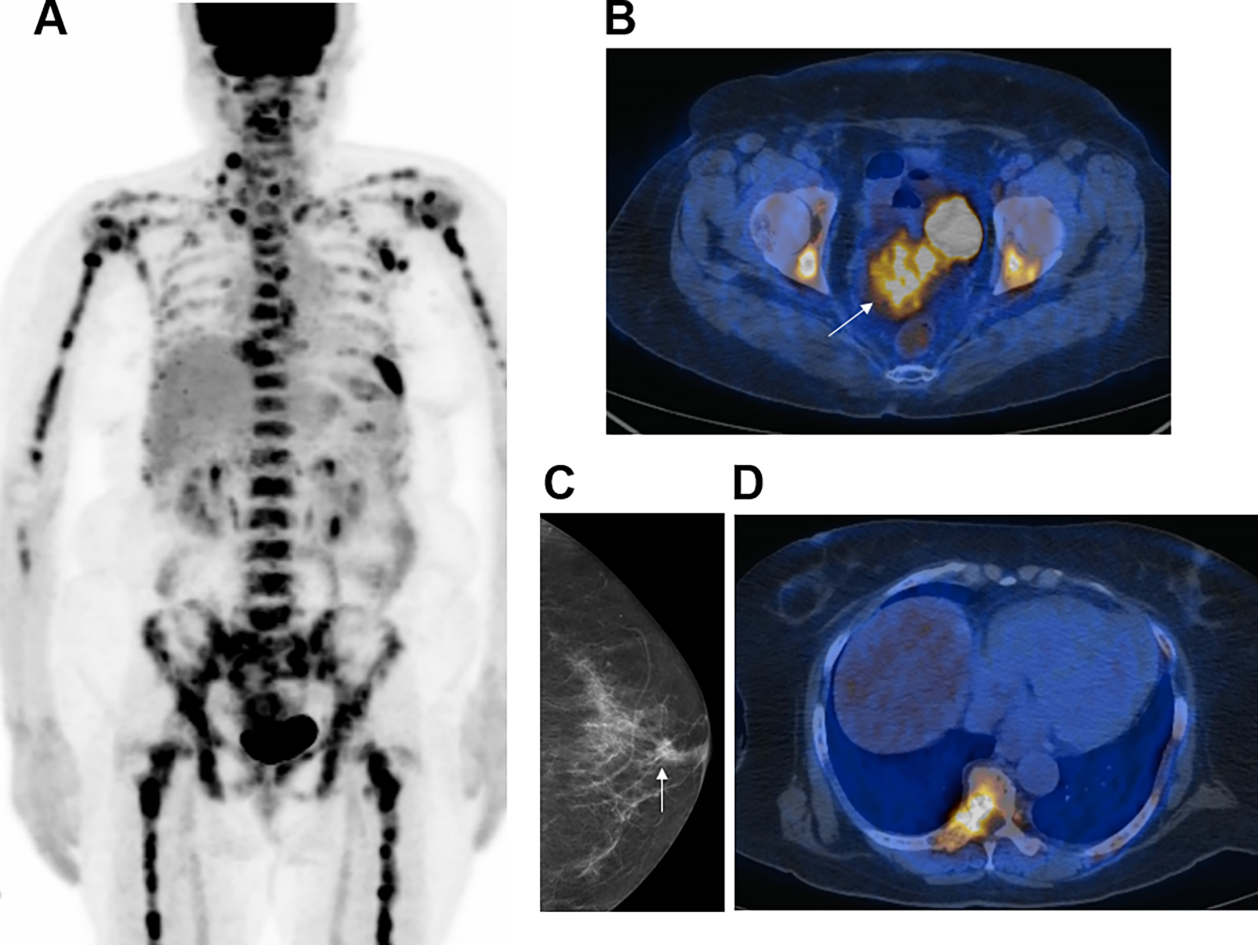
A 65-year-old female with breast cancer missed by FDG PET/CT. (a) The maximum intensity projection image shows multiple hypermetabolic lesions in the skeleton and in the axillary, supraclavicular, cervical and mediastinal lymph nodes. (b) A FDG lesion was detected in the uterus that was interpreted as the primary malignancy (arrow). However, endometrial curettage biopsy confirmed metastasis from poorly differentiated carcinoma. (c) Mammography revealed a 0.9 cm subareolar nodule (arrow) (d) that was FDG non-avid on PET/CT. Biopsy confirmed invasive breast carcinoma.
